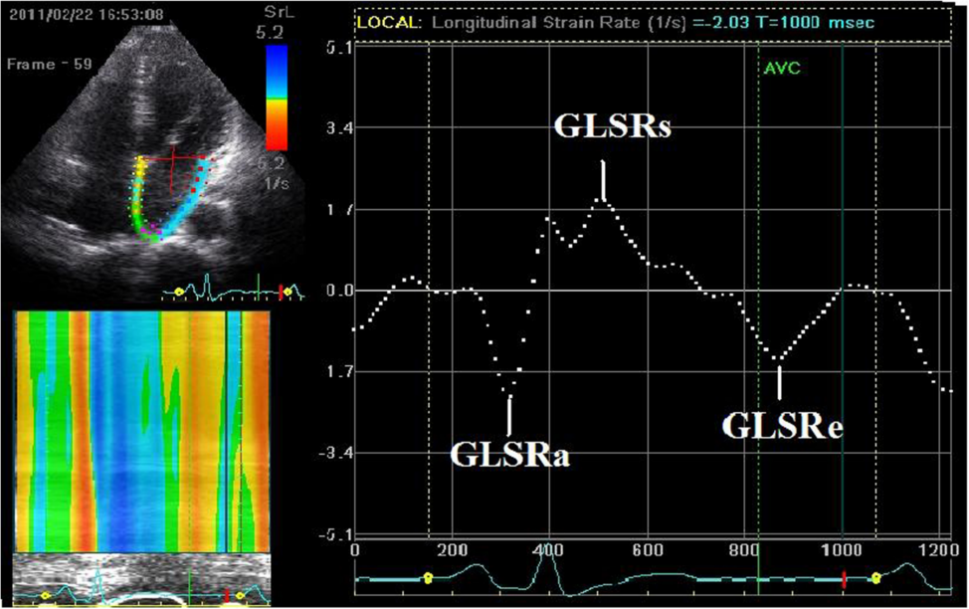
Measurement of global longitudinal left atrial strain rate from an apical four-chamber view. The white dashed curve represents the global longitudinal strain rate during the cardiac cycle. GLSRa = global left atrial peak negative strain rate during late ventricular diastole. GLSRs = global left atrial peak positive strain rate during ventricle systole. GLSRe = global left atrial peak negative strain rate during early ventricular diastole . AVC = aortic valve closure
